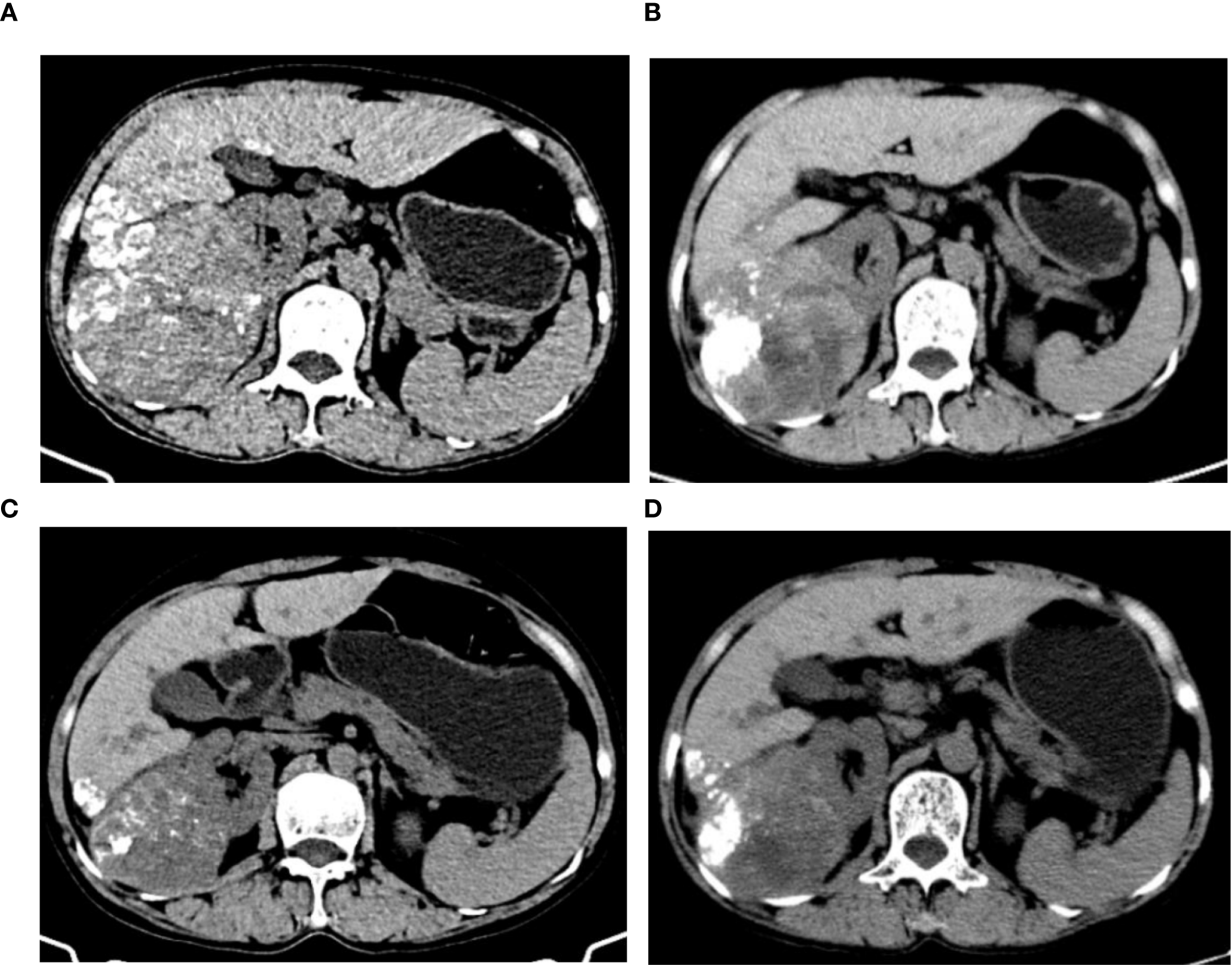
Abdominal CT scans showed a gradual reduction in the size of the liver mass [(a): April 8, 2025: 9.3 cm × 9.1 cm; (b): May 14, 2025: 8.2 cm × 7.3 cm; (c): June 12, 2025: 8.1 cm × 6.7 cm; (d): July 26, 2025: 6.2 cm × 5.9 cm].
